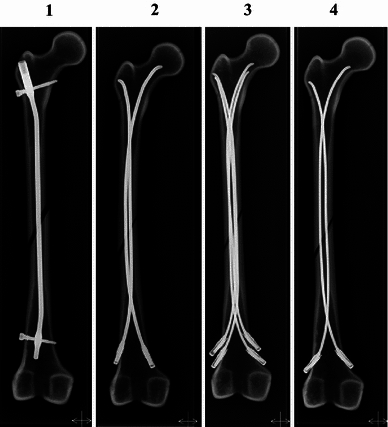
Frontal radiographs of femur models after fixation. 1 One 5.5-mm pediatric locking nail (PLN), 2 two 4.0-mm titanium elastic nail (TEN) with end cap, 3 four 3.0 mm TEN with end caps, 4 two 3.0 mm TEN with end caps
